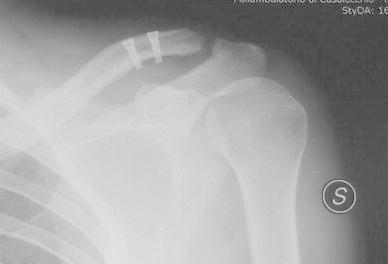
Postoperative X-rays: left AC joint stabilized with the synthetic ligament (LARS LAC^®^)
